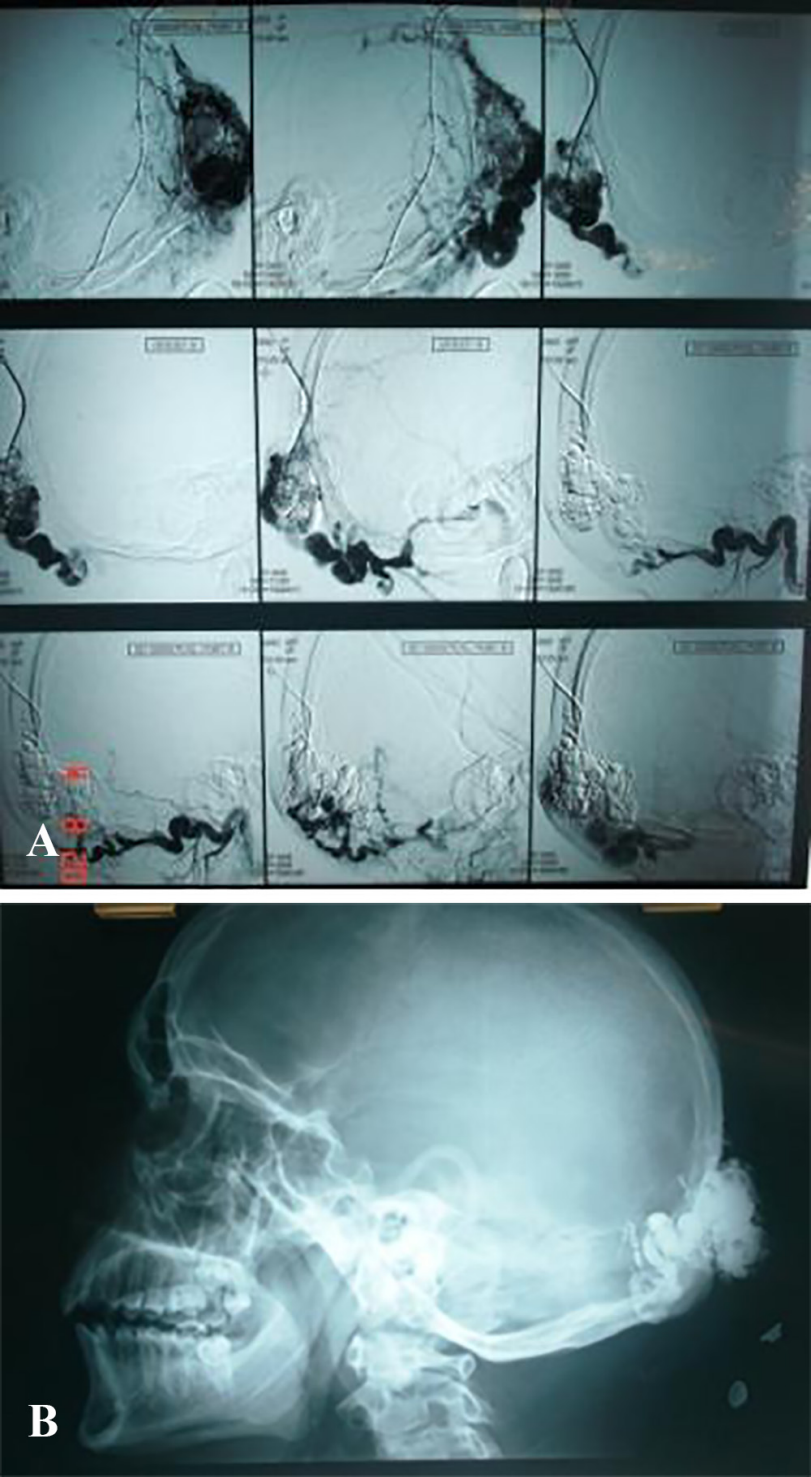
A: Glue embolization of nidus using direct puncture. B: X-ray of skull with nidus embolized prior to surgical excision
